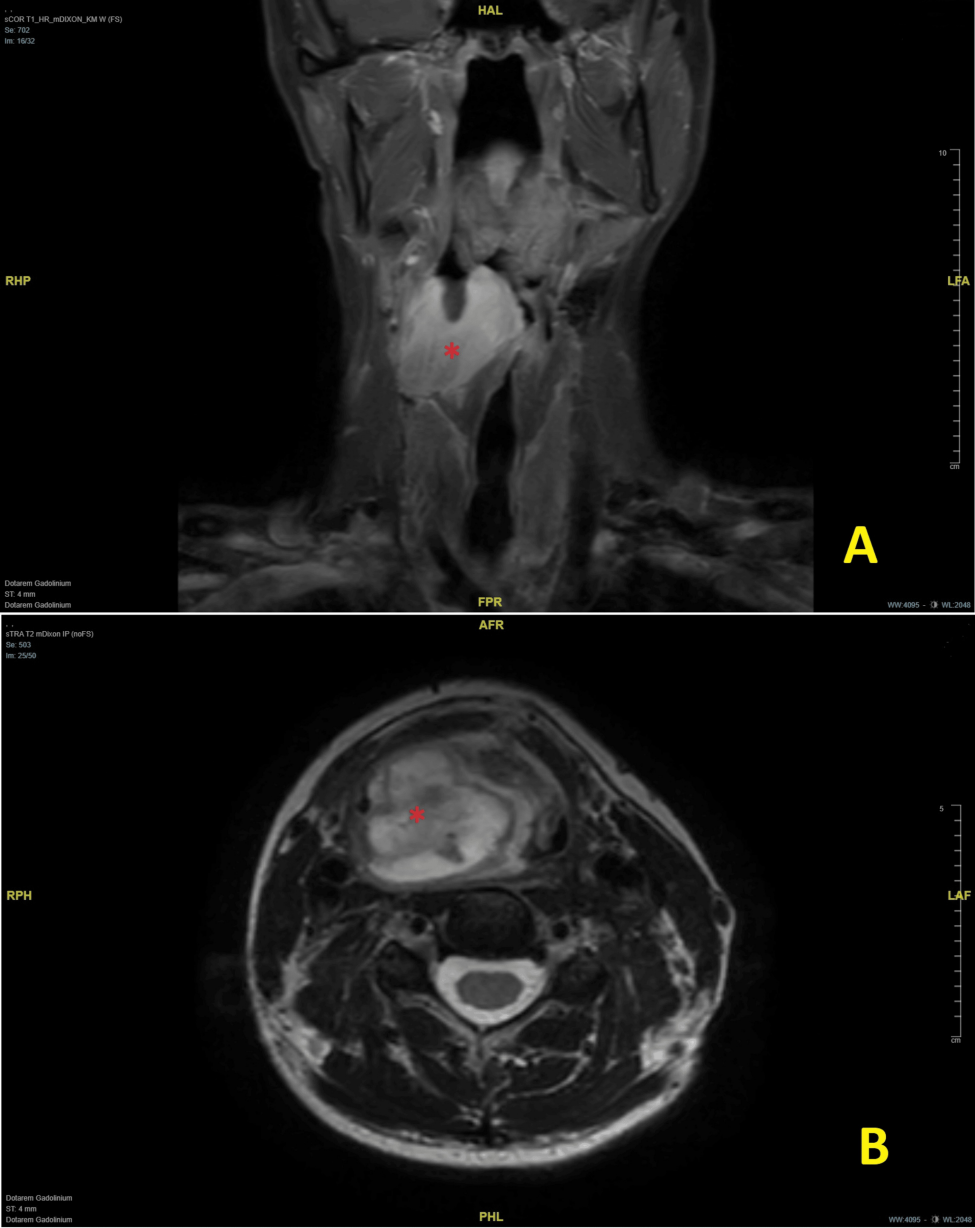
Revised planning MRI. (A) Coronary view, T1 weighted; (B) axial view, T2 weighted. Short-term rapid tumor growth of the partially resected mass (*) in the pyriform sinus.
